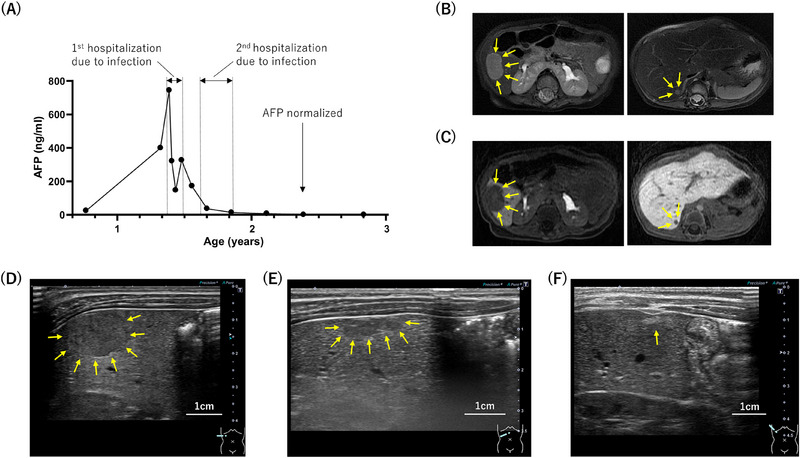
(A) Changes in serum alpha‐fetoprotein (AFP) levels. (B) T2‐weighted magnetic resonance and (C) hepatobiliary phase images with gadolinium‐ethoxybenzyl‐diethylenetriaminepentaacetic acid enhancement displaying tumors located in Couinaud segments 5 (left) and 7 (right) at 1 year and 2 months of age. (D–F) Hepatic ultrasonography of the tumor in the Couinaud segment 5 at 1 year and 5 months, 1 year and 10 months, and 2 years and 9 months of age, respectively. The tumor gradually decreased, with only a residual scar at 2 years and 9 months of age.
